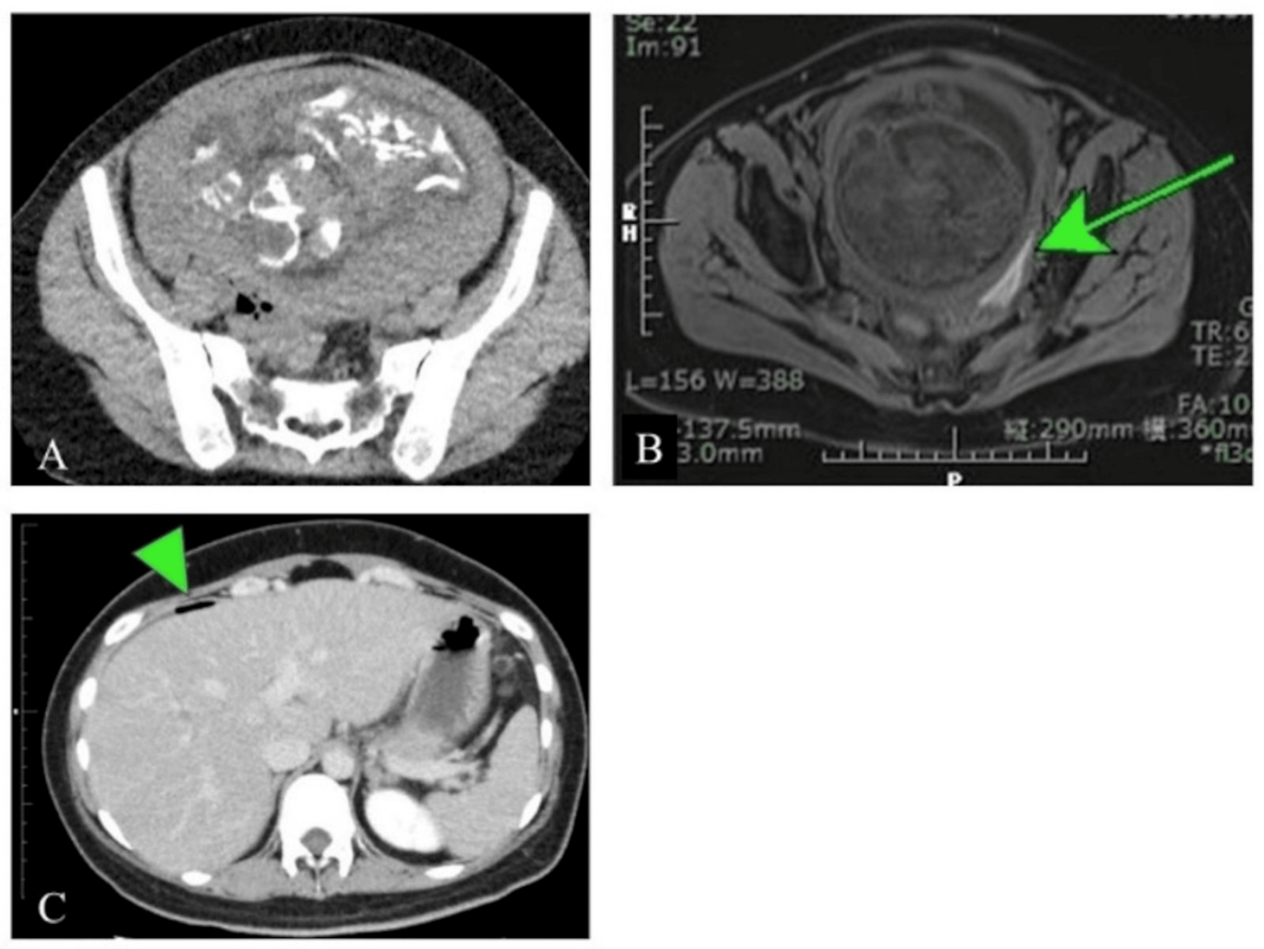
Abdominal computed tomography (CT) and magnetic resonance imaging (MRI) at 31 gestational weeks. (A) Plain CT image at 31 weeks and 1 day. The image shows no evidence of acute appendicitis. (B) T1-weighted MRI at 31 weeks and 2 days. The image reveals hemorrhagic fluid accumulation in the pouch of Douglas (arrow). (C) Contrast-enhanced CT image at 31 weeks and 2 days. The image reveals free air under the right diaphragm (triangle) with no evidence of acute appendicitis or peri-appendiceal free air.
